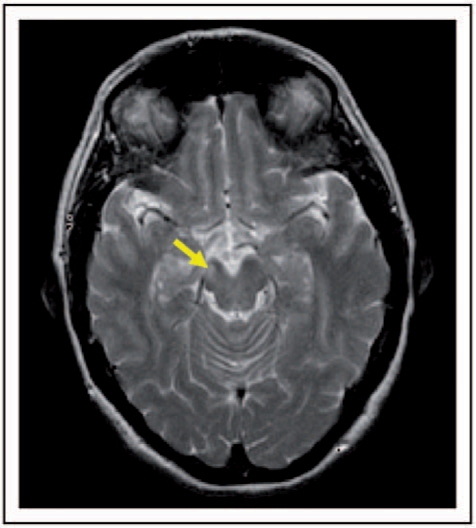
Axial T2-weighted MRI in a patient. The arrow indicates a lesion in the right corticospinal tract (CST).
